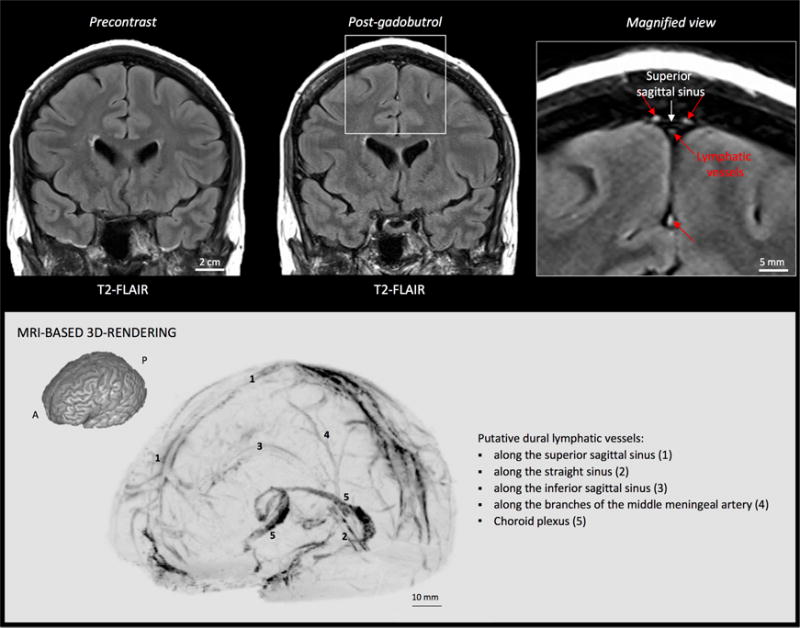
MRI visualization of dural lymphatic vessels in human On post-gadobutrol coronal T2-FLAIR, the dura does not enhance, and lymphatic
